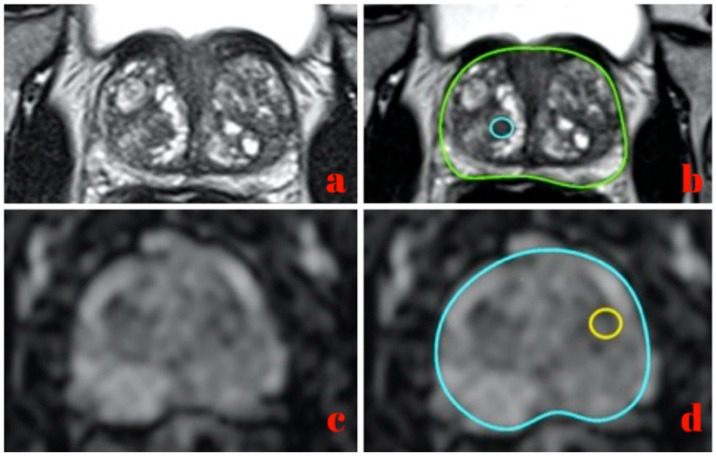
Image segmentation on a T2w MRI slice (a,b) and ADC MRI slice (c,d).
